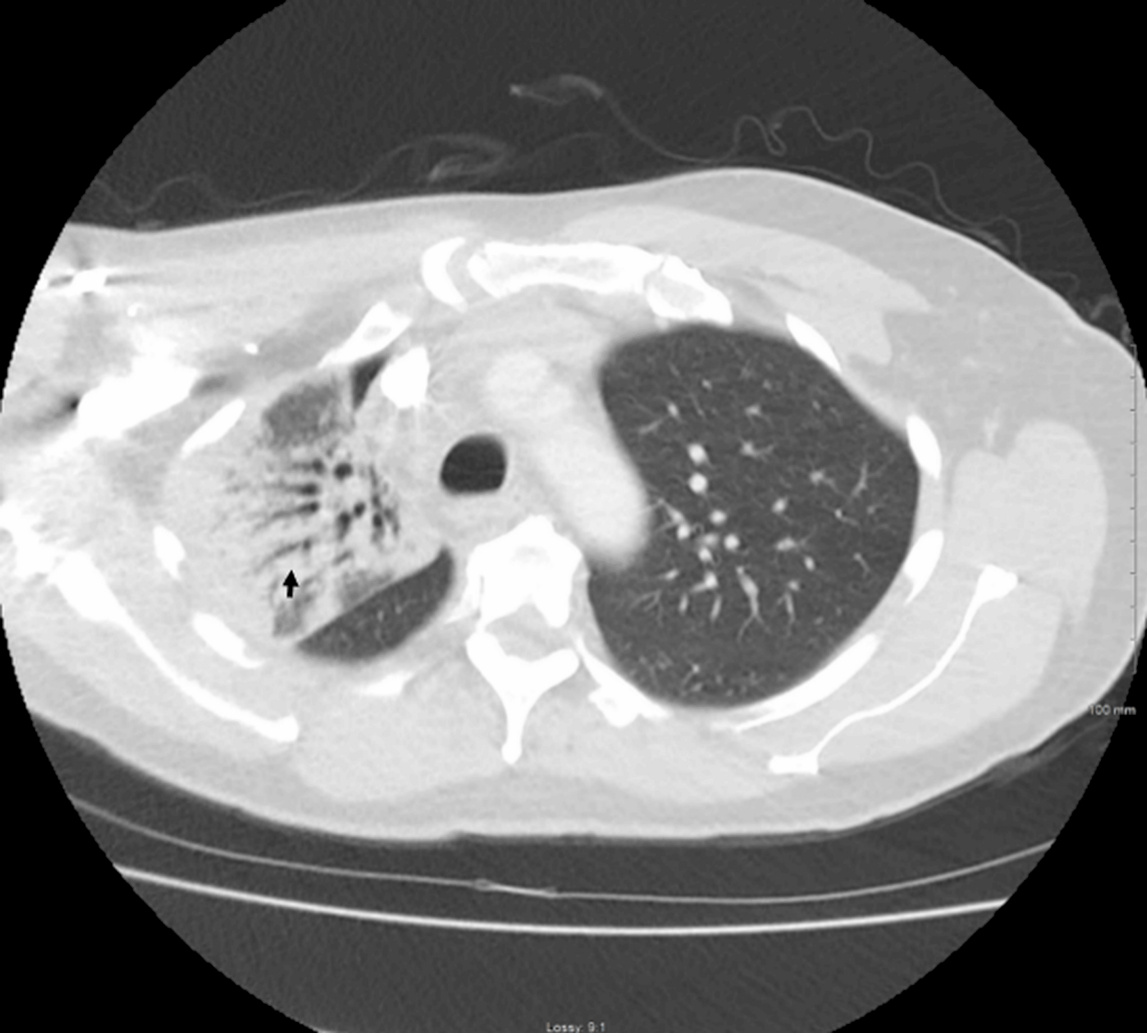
Transverse chest CT image demonstrating significant resolution of the right upper lobe infiltrative changes following treatment (arrow).
